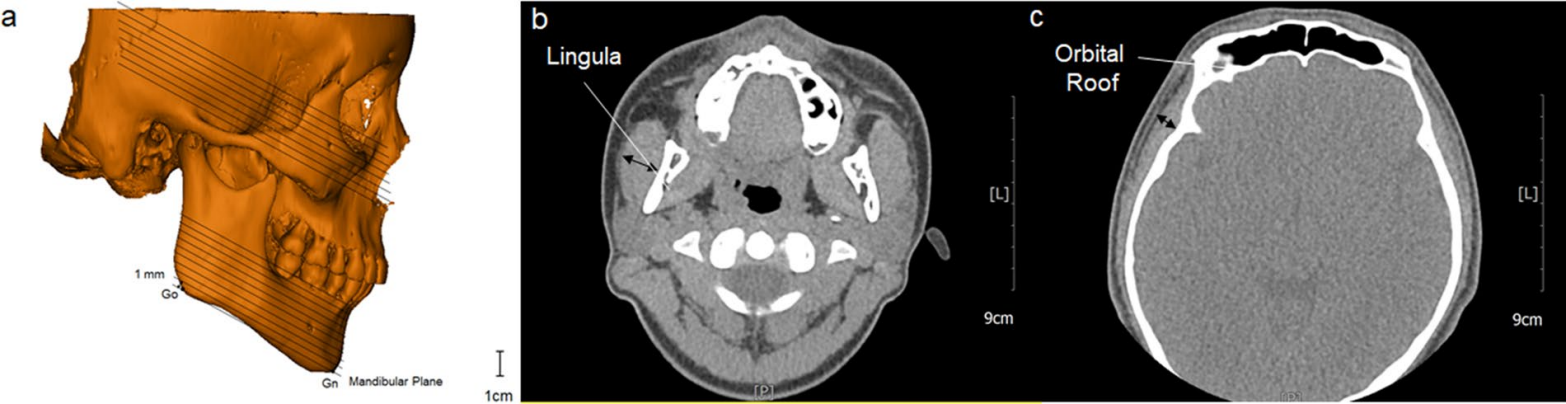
(a) The TMJ CT images were digitally cut into 1 mm thick sections that were parallel to the mandibular plane. (b) The maximum thickness of the masseter muscles were estimated on the sectioning plane, which passes through the lingula (c) The maximum thickness of the temporalis muscles were estimated on the sectioning plane, which passes through the orbital roof.
